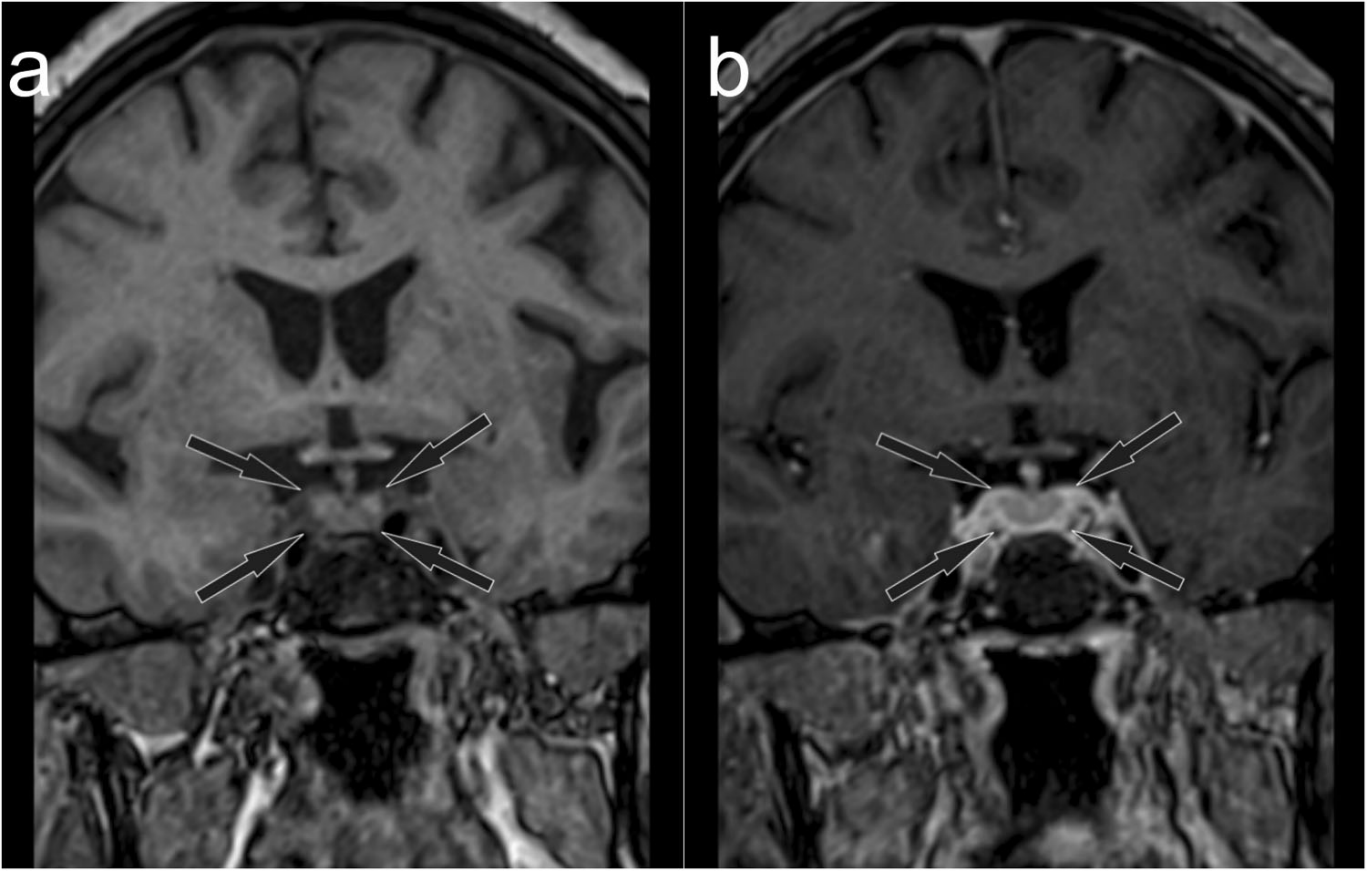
Follow-up MRI scan, coronal plane, before (a) and after (b) contrast medium administration. Normal enhancement of the pituitary gland (marked with black arrows)
